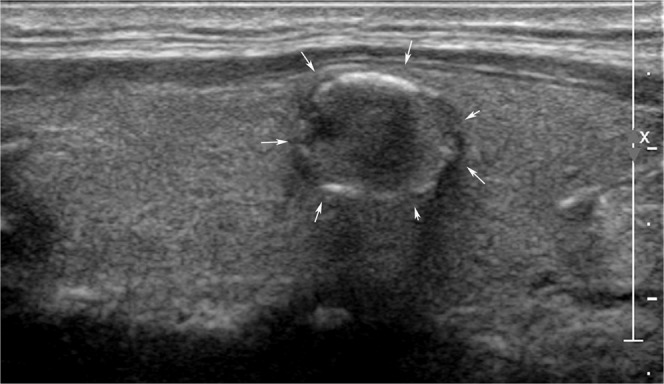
An ultrasonography (US) image of a 50-year-old woman with an incidentally detected thyroid nodule discovered on screening examination that shows a 1.2-cm sized hypoechoic solid nodule with eggshell calcifications (arrows). All 6 radiologists interpreted the nodule as a benign. In contrast, 3 CNN-combinations interpreted it as cancer. The nodule was diagnosed as papillary thyroid cancer by surgery.
